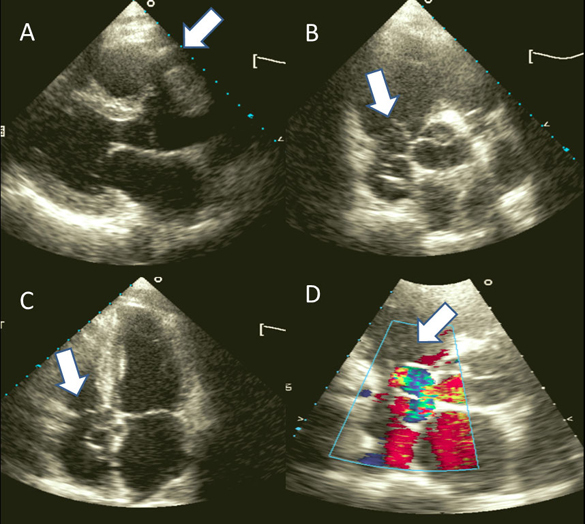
Transthoracic echocardiogram performed showed the tubular structure coming off the right coronary cusp (bold arrow, image A); saccular formations adjacent to the aortic root in short axis and apical 4 chamber view (bold arrows, image B and C); as well as predominantly diastolic turbulent flow within the saccular formations (image D).*
